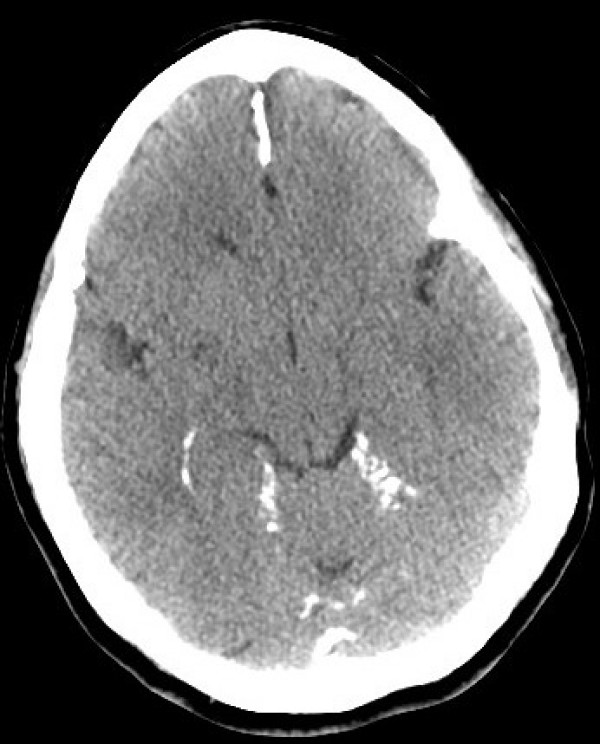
Unenhanced brain computed tomography scan (Axial view):  Ectopic multiple gross calcifications of falx cerebri are visible.
